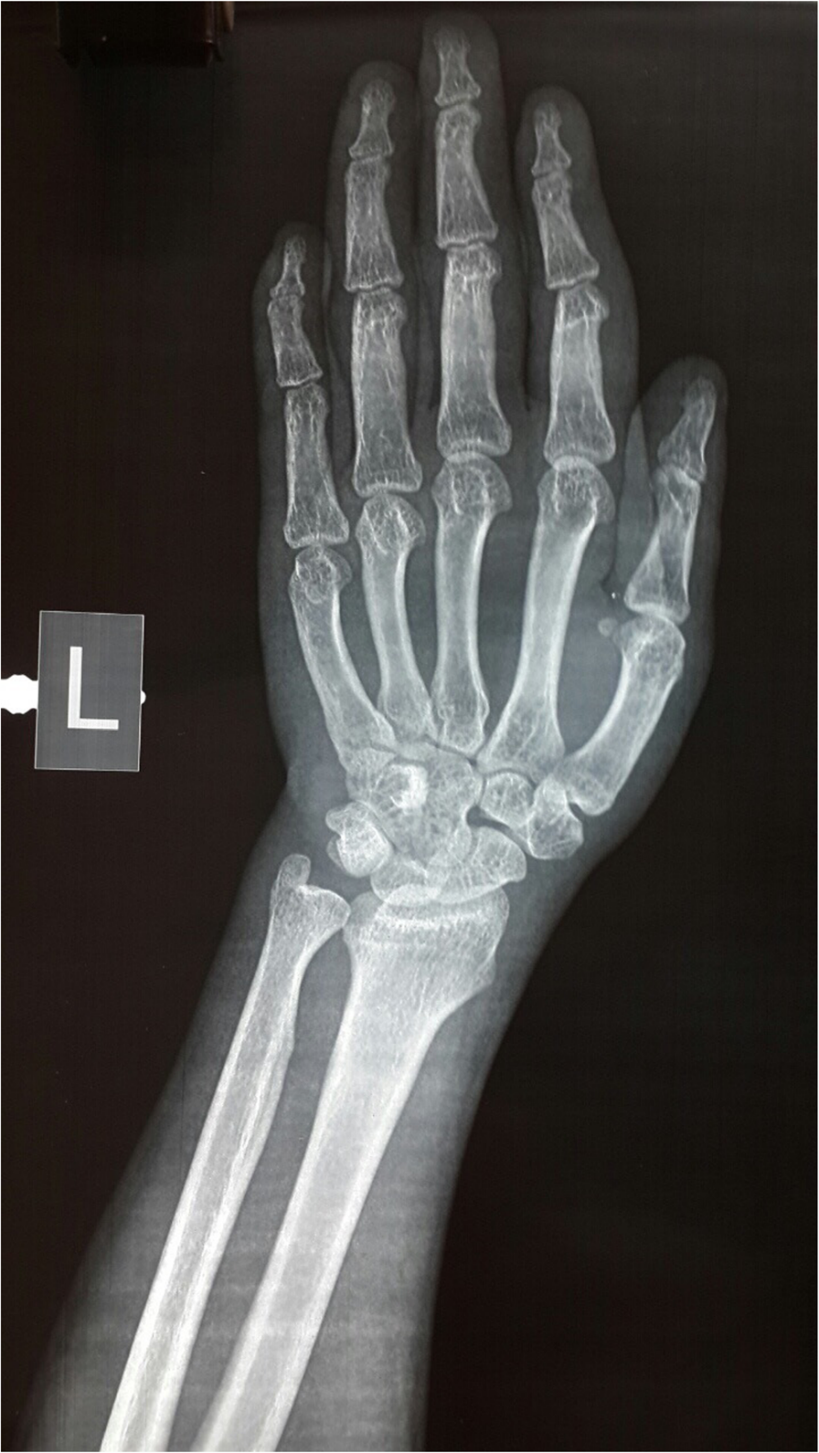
X-ray of left hand showing epiphyseal fusion. The lower end of the radius shows epiphyseal closure and ulna also shows epiphyseal closure (bone age is intermediate between 17 and 18)
